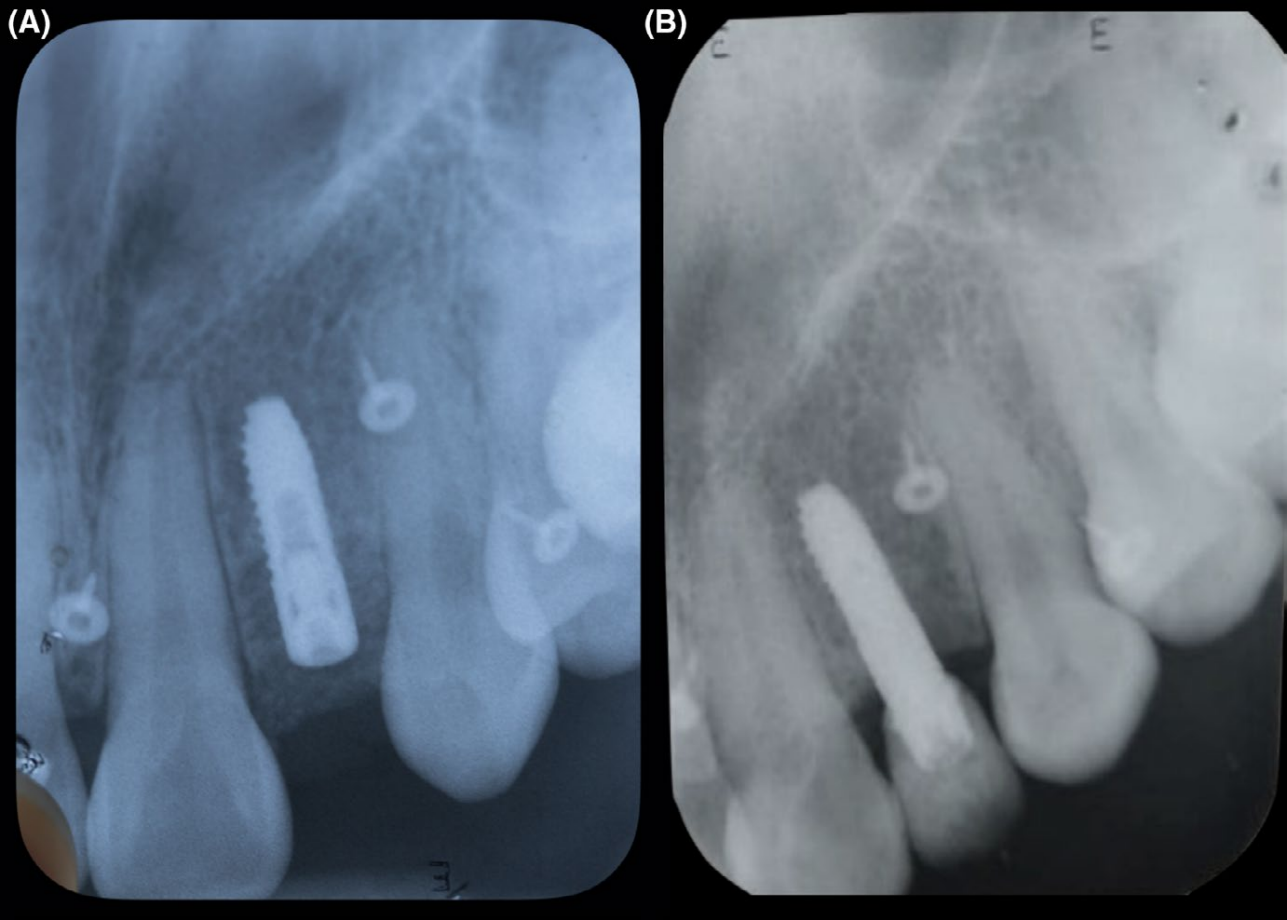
(A) Radiograph: initial placement. (B) Radiograph: 4 years follow‐up
